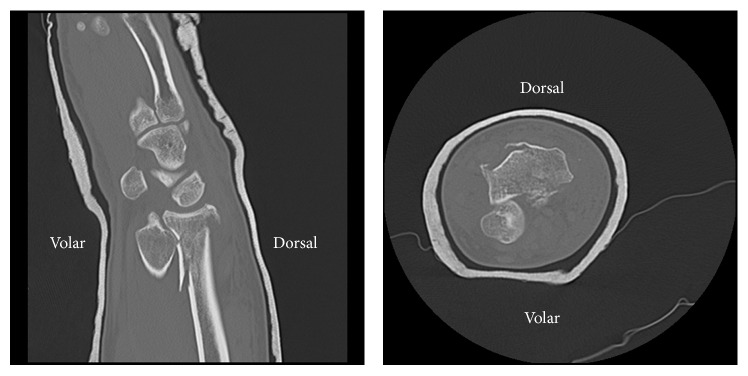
Sagittal and coronal computed tomography views showing the volar DRUJ dislocation.
